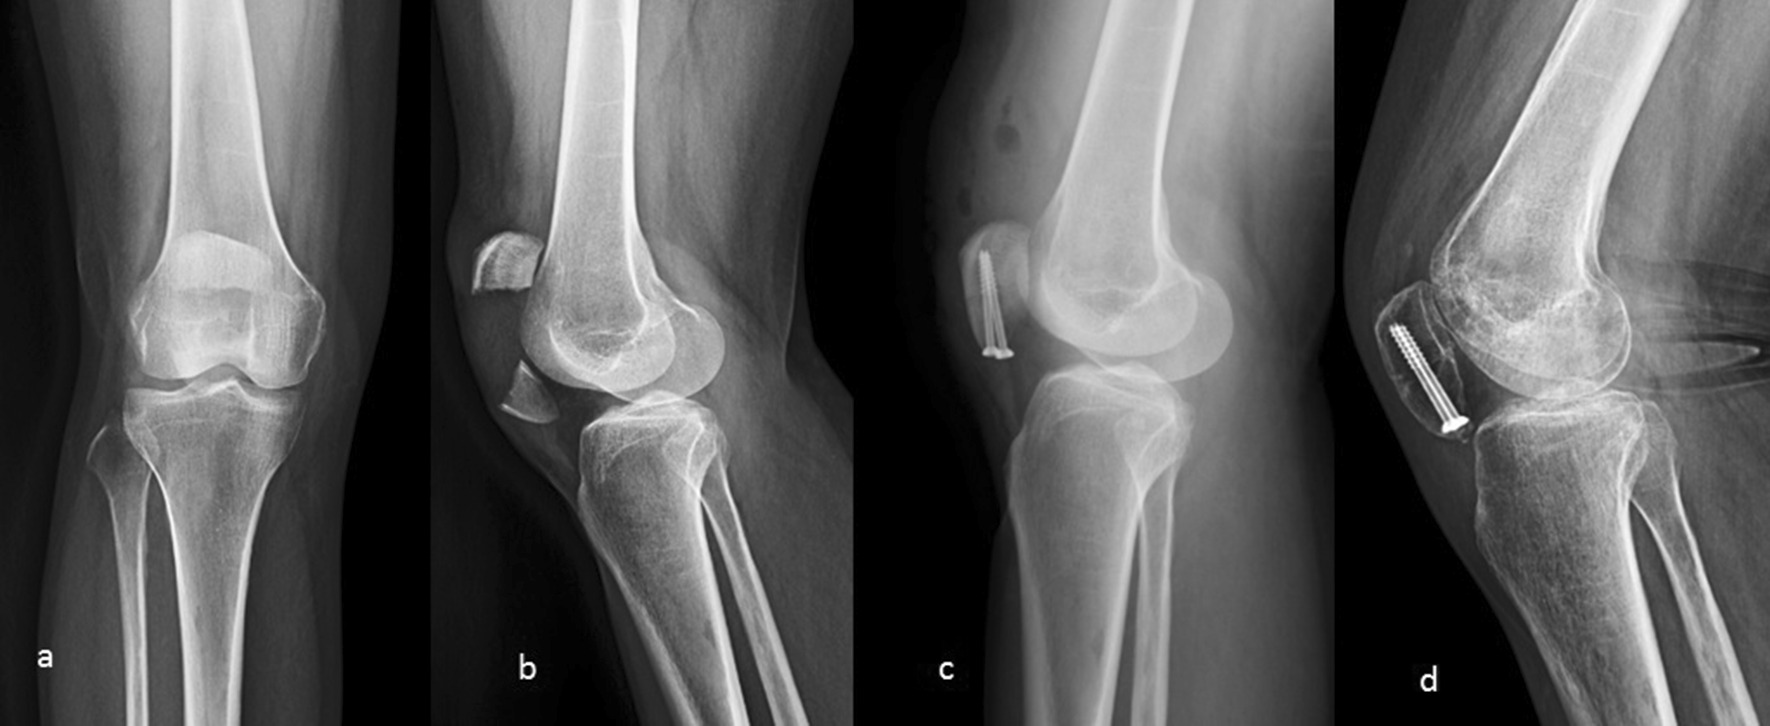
Plain radiographs of the patient (number 23). a before surgery; b before surgery; c immediately after surgery; d at 1 year postsurgery
